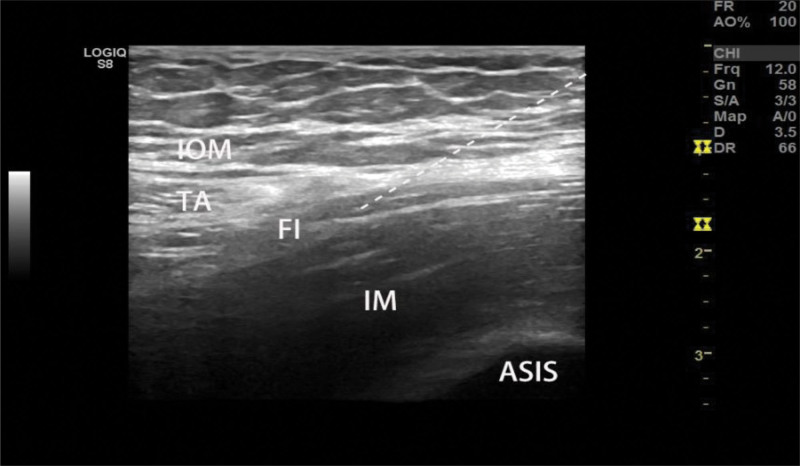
Ultrasonic image of supra-inguinal fascia iliaca compartment block. ASIS = anterior superior iliac spine, FI = fascia iliaca, IM = iliac muscle, IOM = internal oblique muscle, TA = transversus abdominis.
